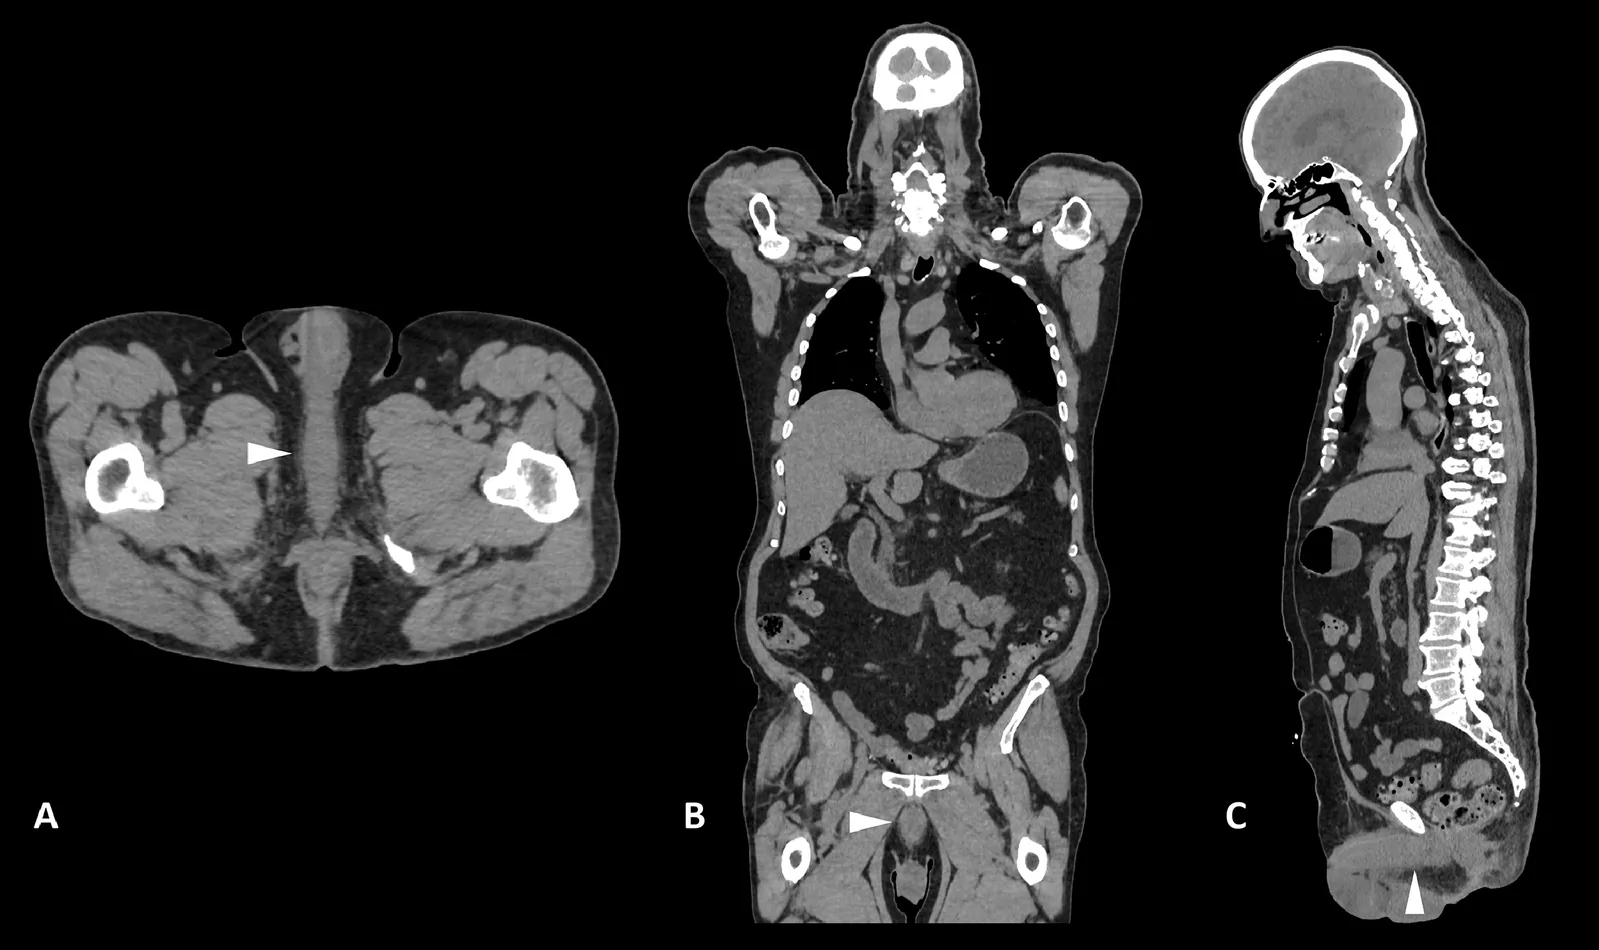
A) Axial B) Coronal C) Sagittal diagnostic CT images did not reveal a lesion to explain the symptoms.
